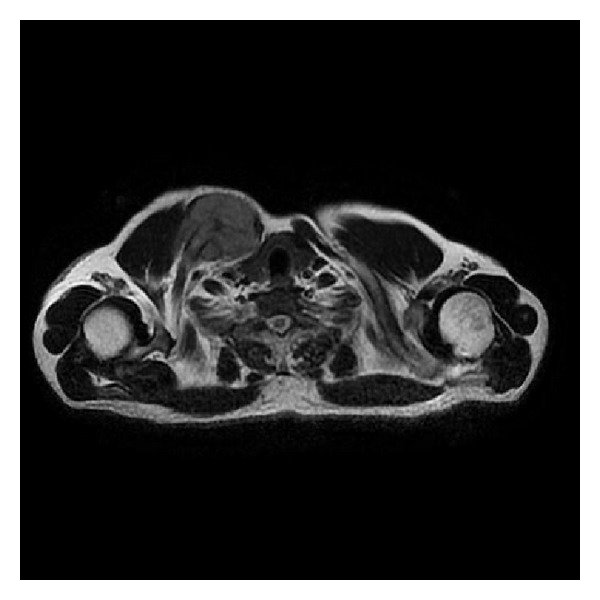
Axial MRI section with mass on the medial side of the clavicle.
